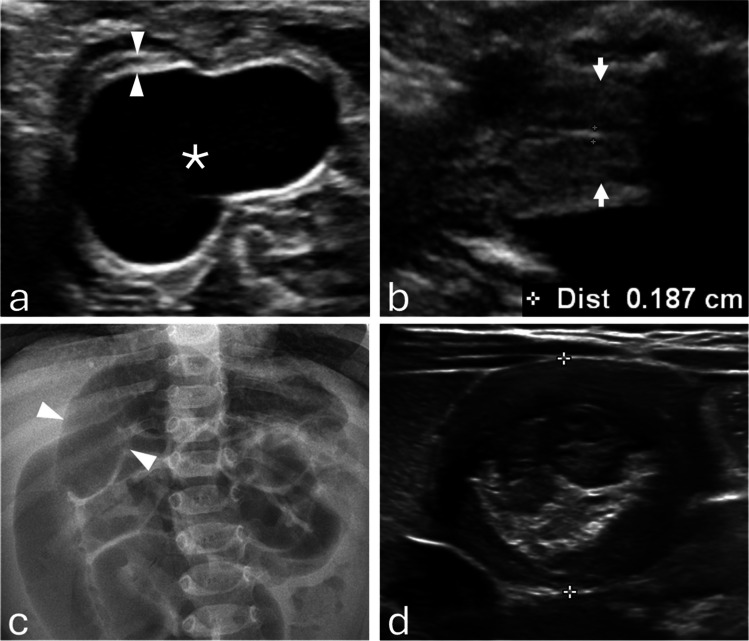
a Prenatal grayscale ultrasound at 34 weeks 3 days demonstrates a pathology-proven enteric duplication cyst (asterisk) with the classic gut signature, consisting of an echogenic inner mucosal layer and a hypoechoic outer muscular layer (arrowheads). b Sagittal grayscale ultrasound shows the fetal uterus (arrows) with an endometrium measuring < 2 mm. c At 4 months of age, frontal abdominal radiograph demonstrates small-bowel obstruction (arrowheads). d Grayscale ultrasound demonstrates an ileocolic intussusception caused by the enteric duplication cyst. The infant underwent surgical resection
